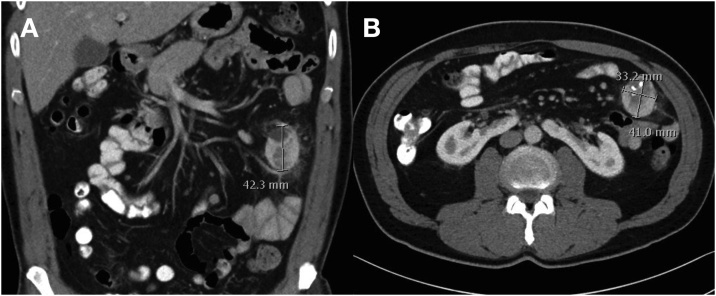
CT scan with suspicious finding at the mesentery. In the mesentery with close contact to the small intestine, we detected a both, cystic and calcified area with a size of 4.2 × 4.1 × 3.3 cm. In addition, strong contrast enhancement and imbibed perifocal adipose tissue, as well as suspicious swelling of the perifocal lymph nodes was described. As a secondary finding, a horseshoe kidney resulted, otherwise no interesting findings. Images in coronary (A) and axial alignment (B). CT scan is enhanced by oral, rectal and intravenous applicated contrast agent (iodine based).
